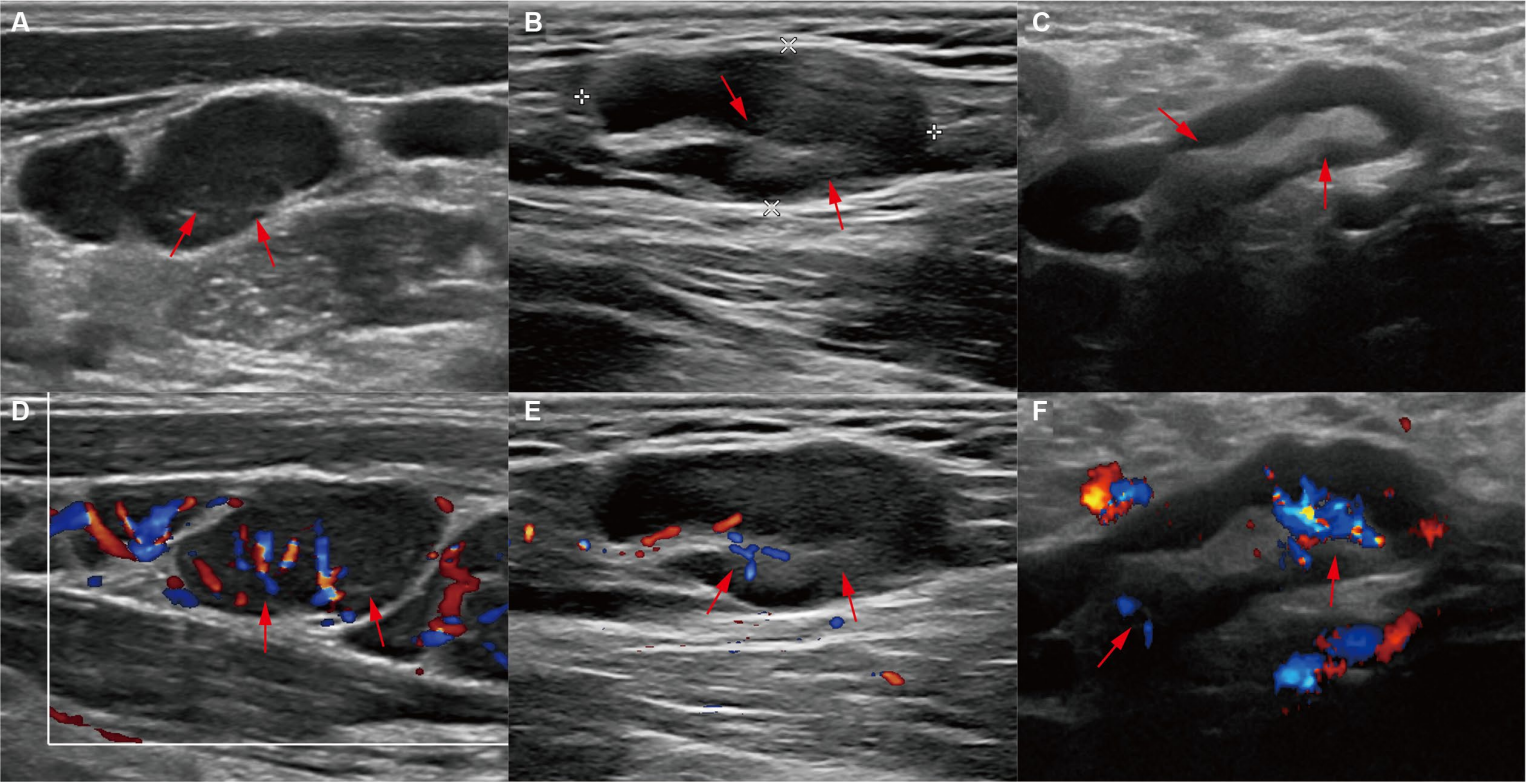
Ultrasound features of present or thinned hila. (A) Ultrasound images and (D) color Doppler flow imaging (CDFI) of the left cervical lymph node (LN) from a 34-year-old patient showed a thinned hilum and a hilar-type blood flow signal (red arrows). (B) Ultrasound images and (E) CDFI of the left cervical LN from a 31-year-old patient showed a thinned hilum and a hilar-type blood flow signal (red arrows). (C) Ultrasound images and (D) CDFI of the left axillary LN from a 53-year-old patient showed a present hilum and a hilar-type blood flow signal (red arrows).
